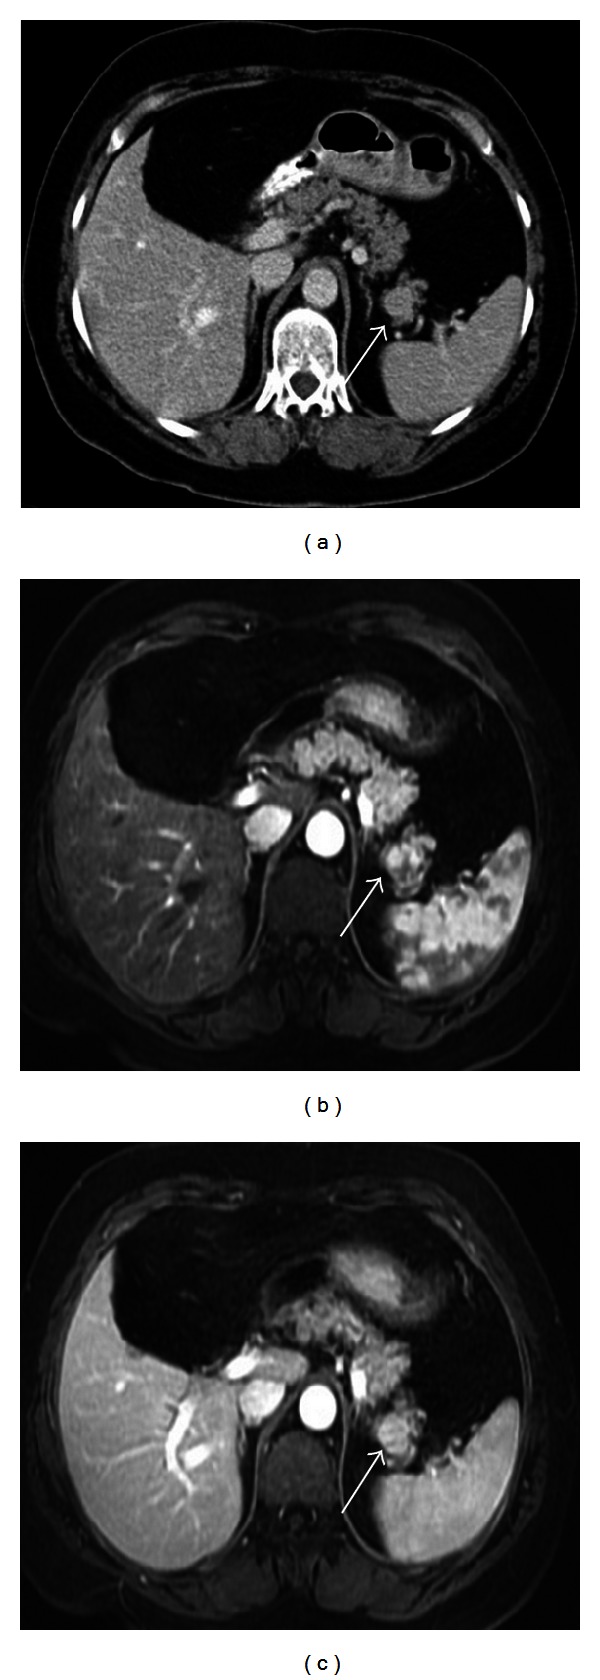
Axial contrast enhanced CT image (a), contrast enhanced T1-weighted image at arterial (b) and venous phases (c) show an intrapancreatic nodular mass in a 63-year-old female patient. The mass has similar density at CT and contrast enhancement pattern at MR images with the spleen indicating an intrapancreatic accessory spleen.
